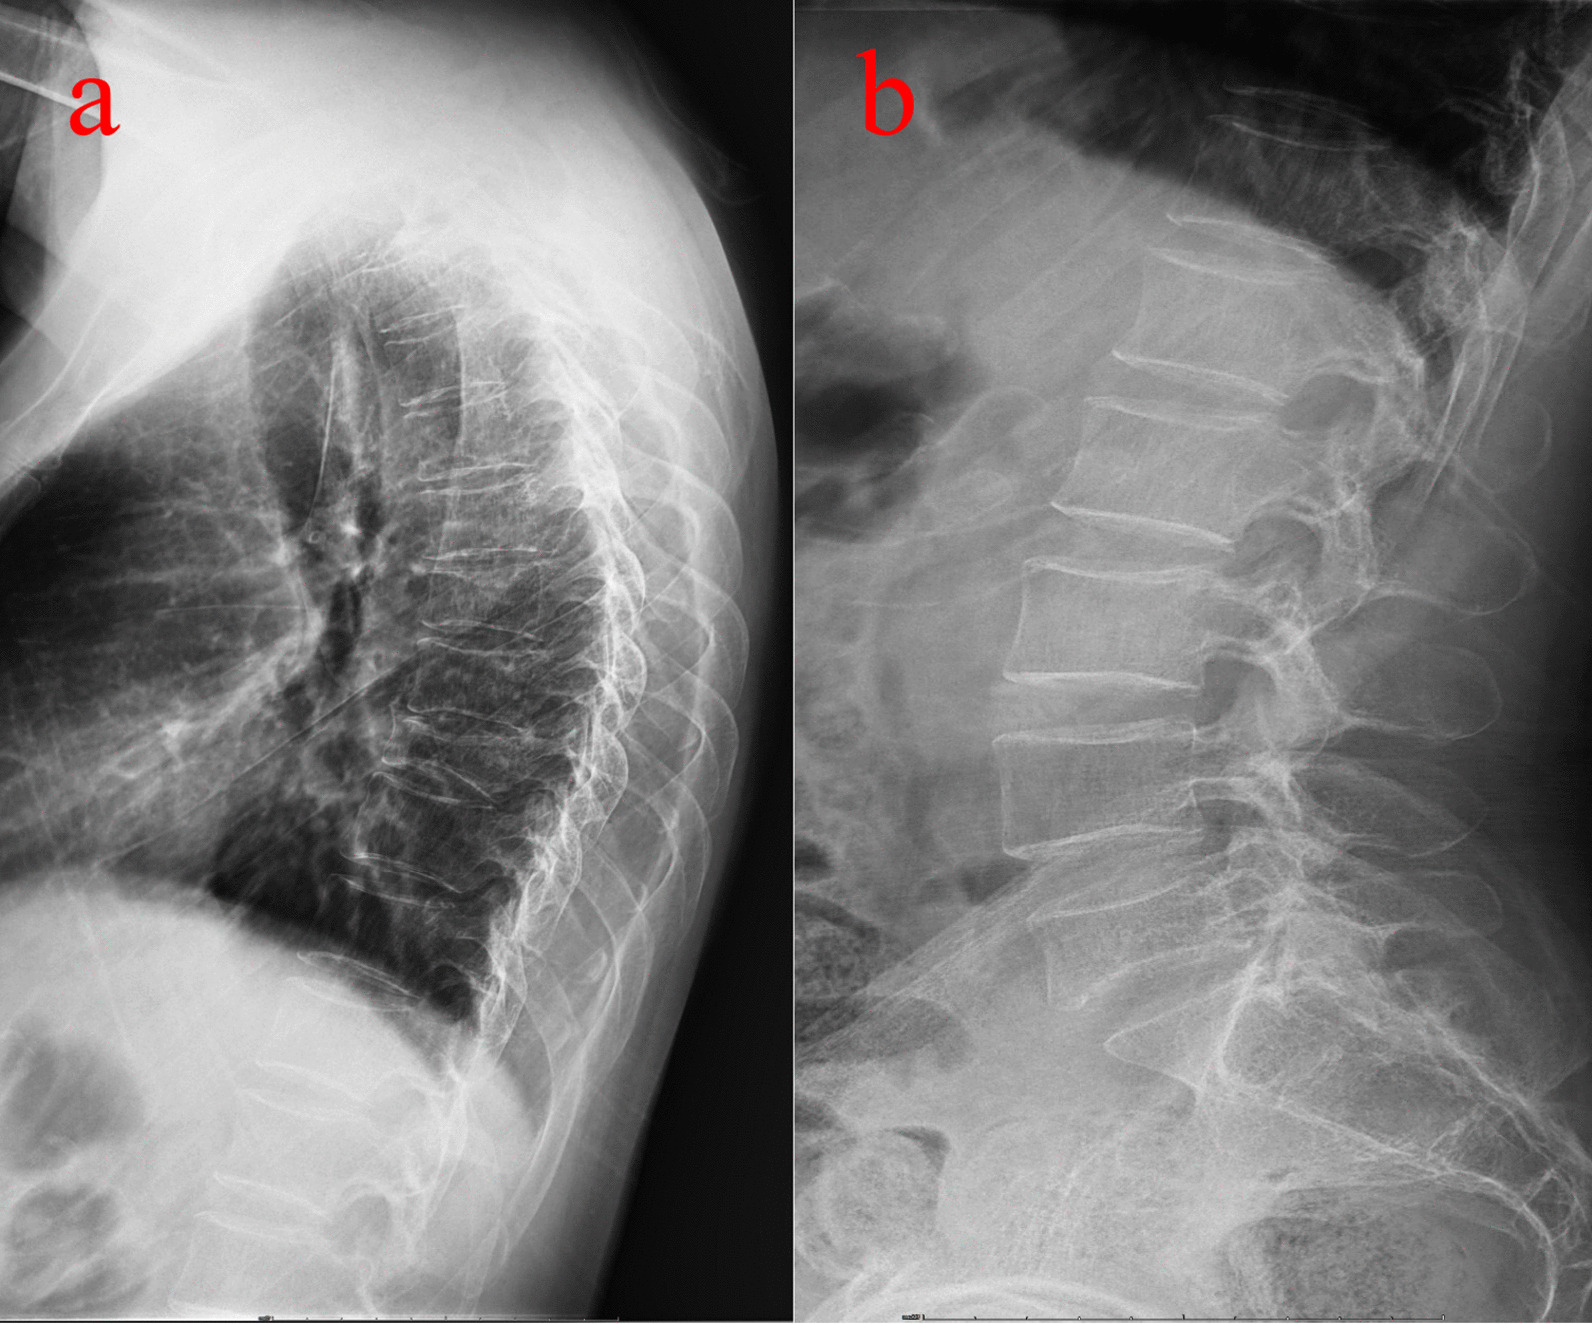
Radiology date of the patient at 1-year follow-up. a The lateral X-ray showed showed T9. b The lateral X-ray showed L5
